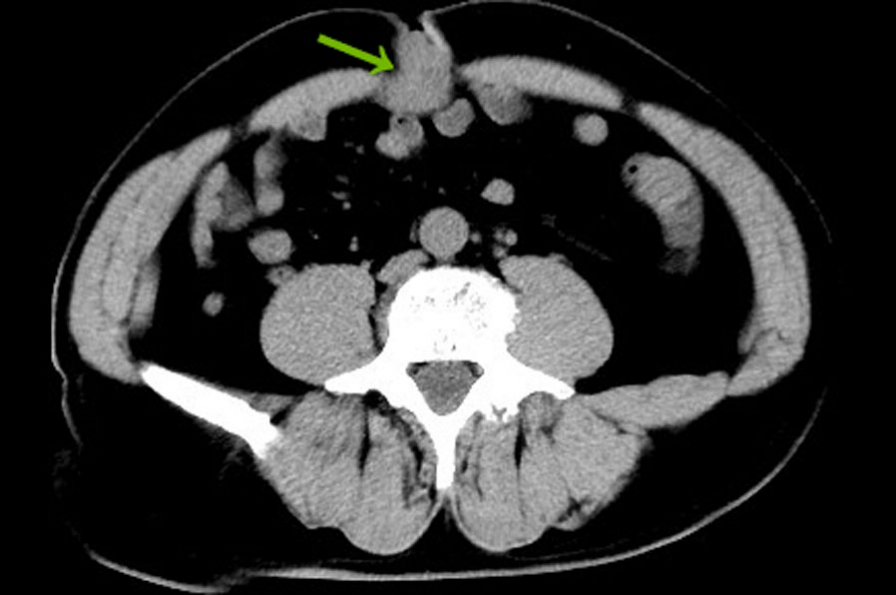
Abdominal CT scan: umbilicus lump (*arrow*), which was suspected to be an umbilicus tumor
